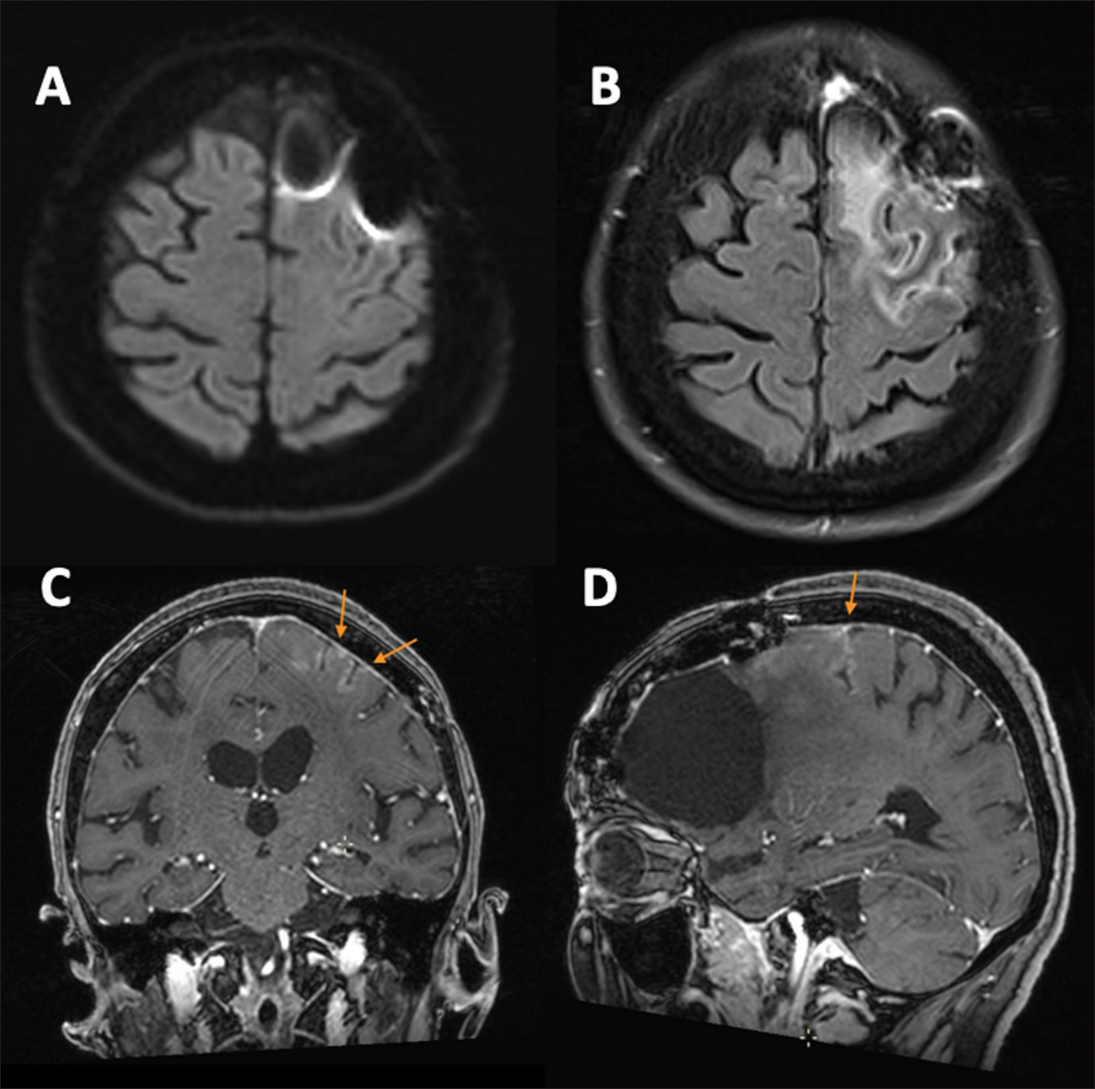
Brain MRI at presentation with no evidence of restriction of diffusion (A). Marked left posterior frontal gyral edema on axial FLAIR (B) associated with cortical gyriform gadolinium enhancement on coronal and sagittal T1-weighted (C–D).
